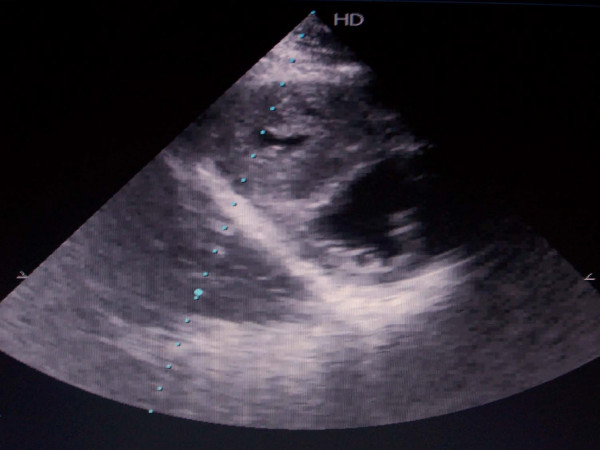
Echocardiography -subcostal view: hypertrophic right ventricule.
